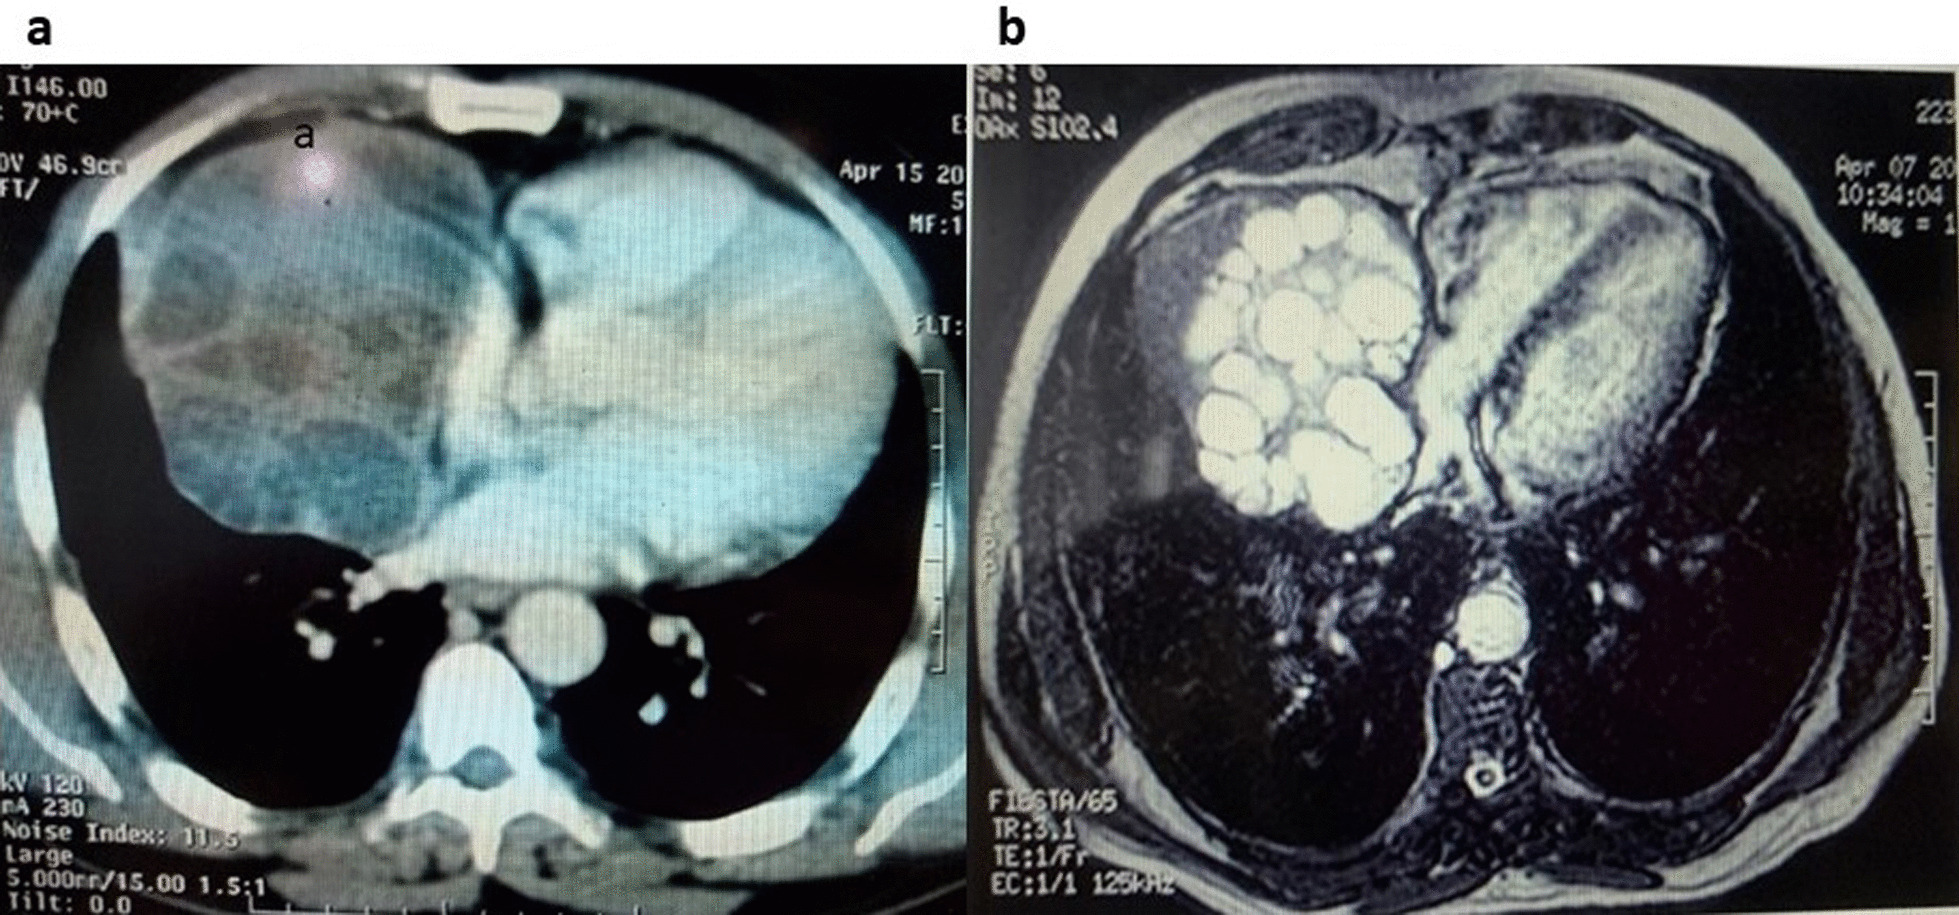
a CT scan established a 11 × 7 cm heterogeneous cystic lesion with unclear boundaries with the pericardium compressing the right atrium and the inferior vena cava. b MRI T2-weighted image, showing the pericardial nature of the lesion without involvement of the atrial wall; the lesion is hyper intense on T2 and hypo intense on T1-weighted images
